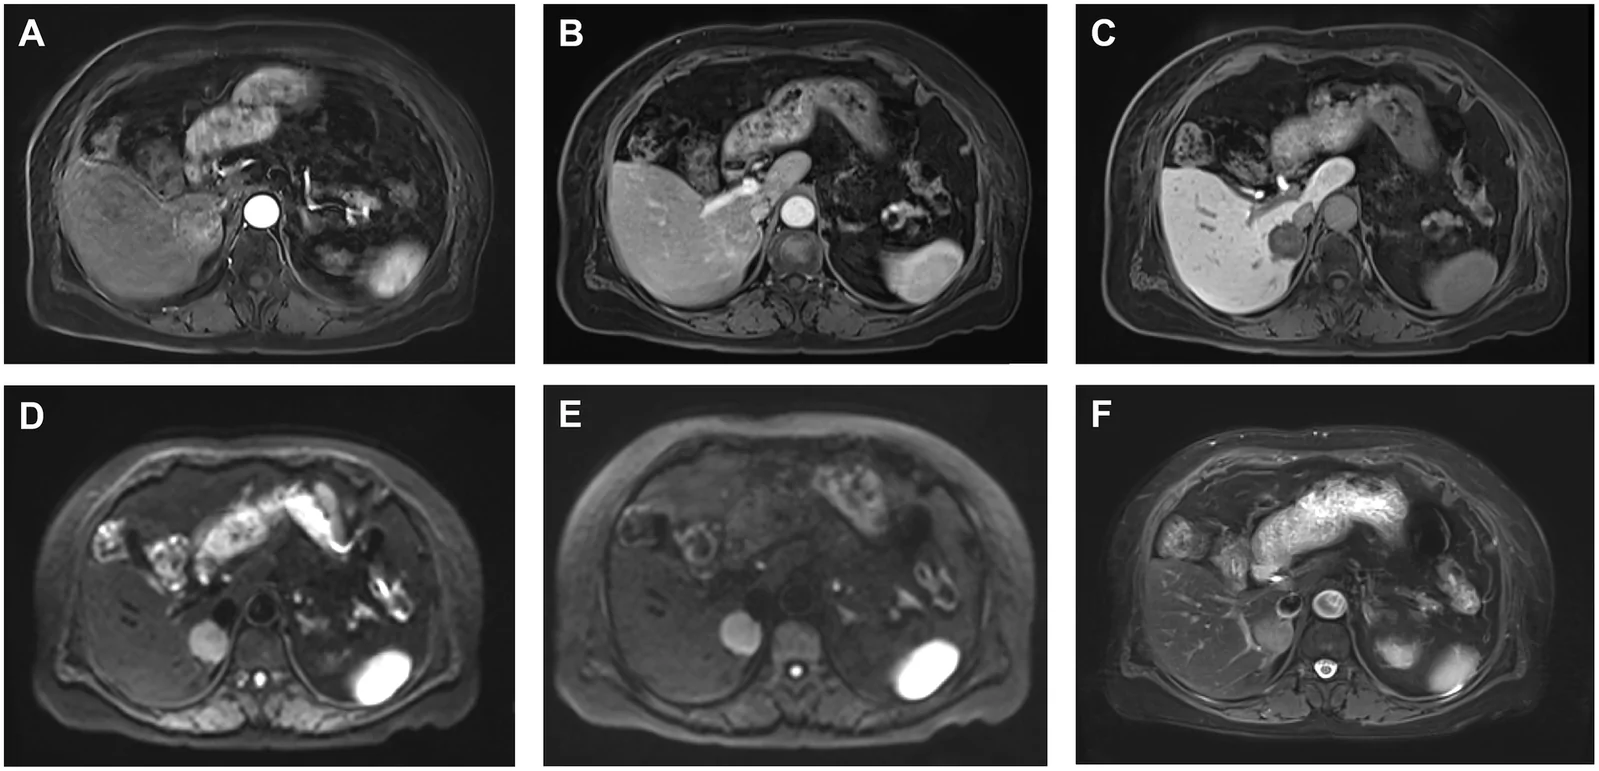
Magnetic resonance imaging findings. (A) Arterial phase image showing marked hyperenhancement of the lesion. (B) Portal venous phase image showing relative hypoenhancement compared with the surrounding liver. (C) Hepatobiliary phase image showing hypointensity of the lesion. (D,E) Diffusion-weighted images showing the lesion in the right posterior liver. (F) Fat-suppressed T2-weighted image showing a mildly hyperintense, well-circumscribed lesion.
